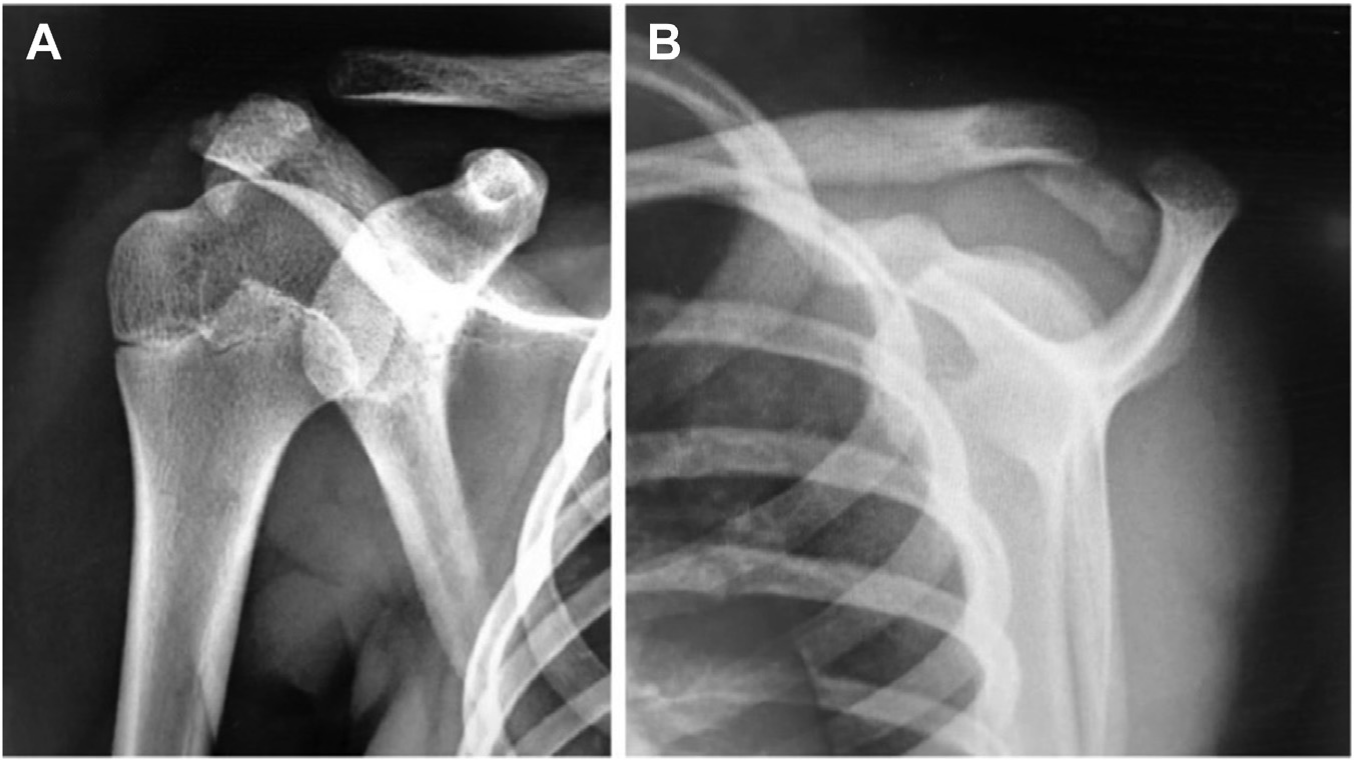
(A) Shoulder anteroposterior and (B) scapular Y radiographs, showing a displaced bony fragment from the acromion, occupying the subacromial space.
